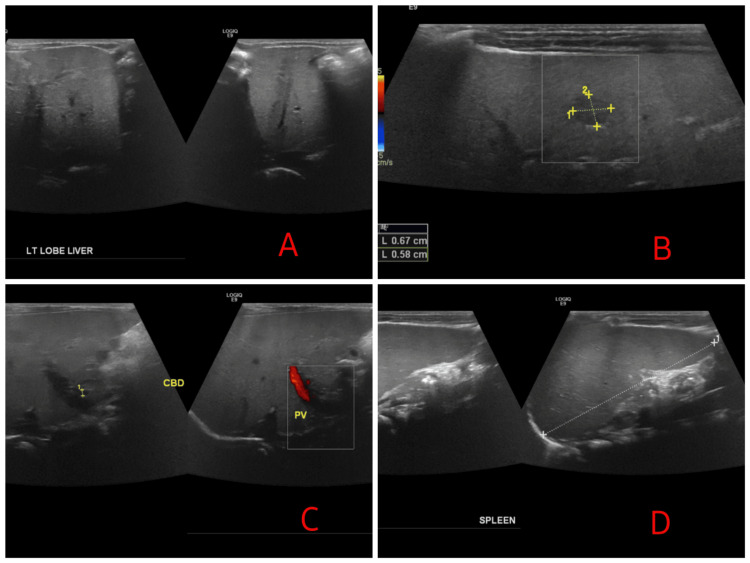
Ultrasound images of the (A) left lobe of the liver, (B) right lobe of the liver, (C) common bile duct, and (D) spleen (A, B) Enlarged liver, with a total span of 87 mm. (B) A hypoechoic lesion 7x5 mm (yellow dots) in the right lobe of the liver. (C) The common bile duct and patent portal vein. (D) Enlarged spleen with a span of 71 mm.
